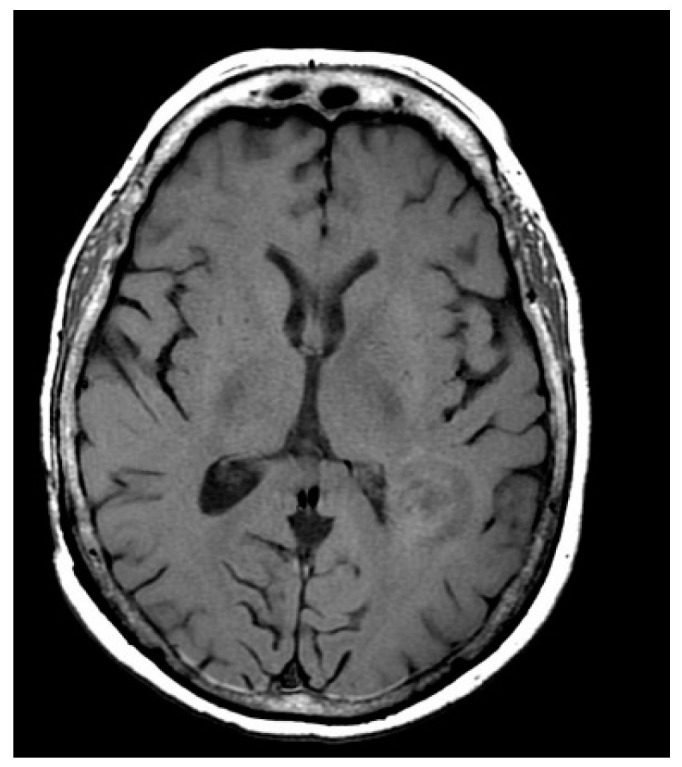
An MRI image with noise.
